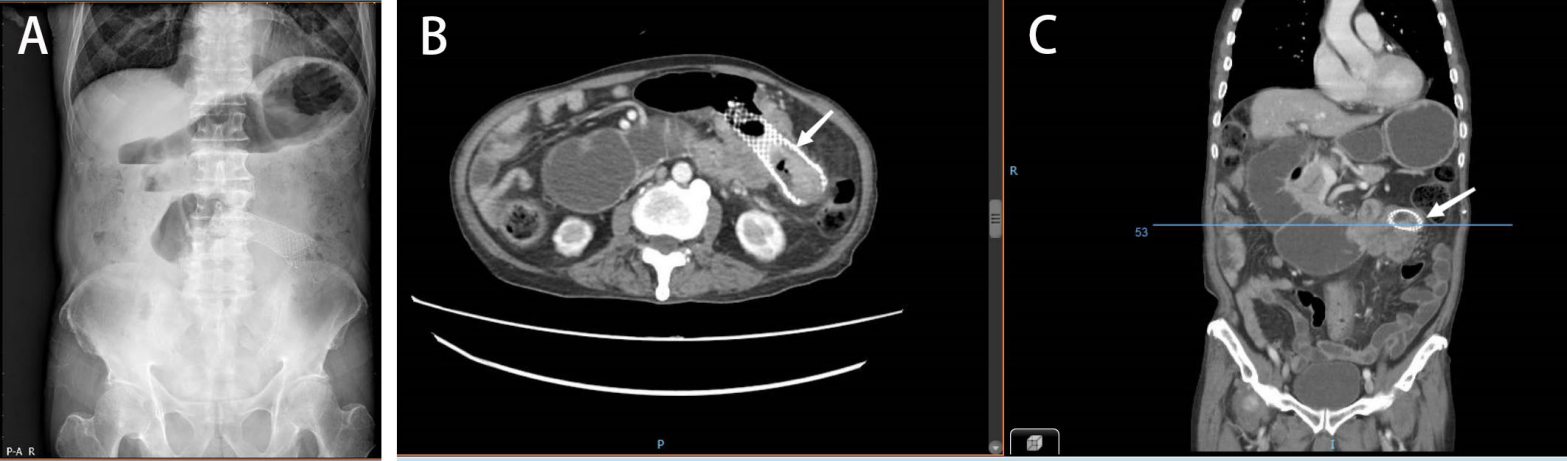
(A) Abdominal plain radiograph showing the duodenal obstruction. (B, C) Abdominal CT demonstrating a tumor invading the duodenum and causing duodenal obstruction due to compression (January 2022).
